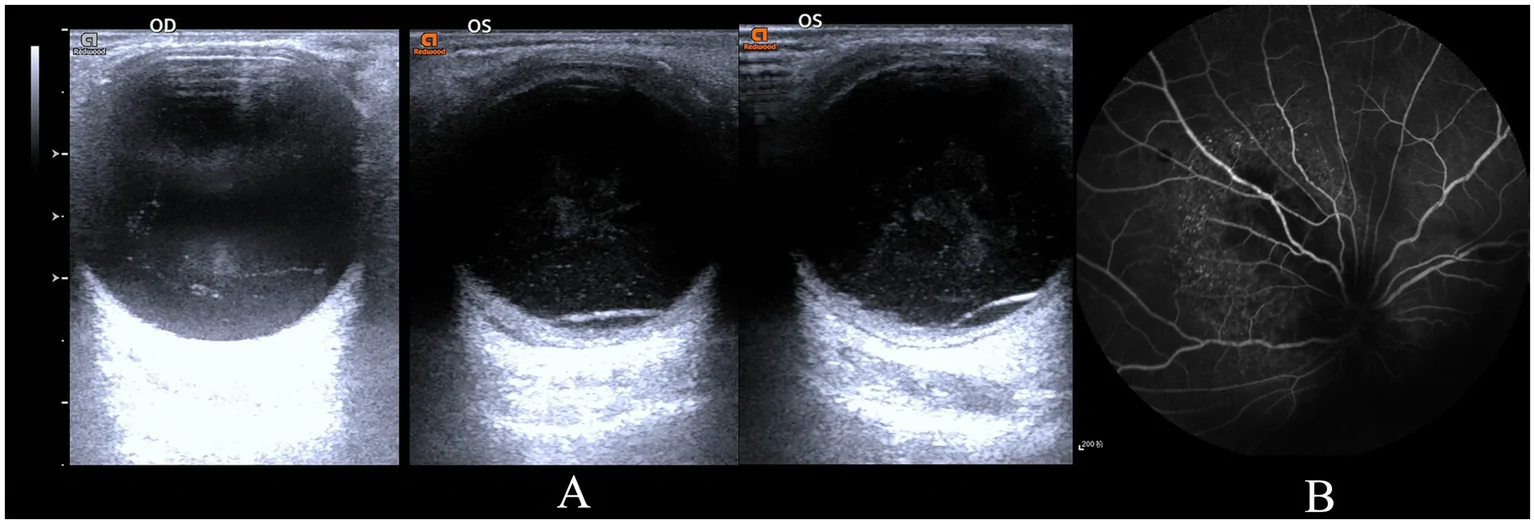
(A) B-scan ultrasonography revealed retinal detachment and posterior scleral thickening in the left eye, along with mild vitreous opacities in the right eye. (B) Fluorescein angiography of the left eye revealed numerous punctate hyperfluorescent areas superonasal to the optic disc, with subsequent dye leakage.
